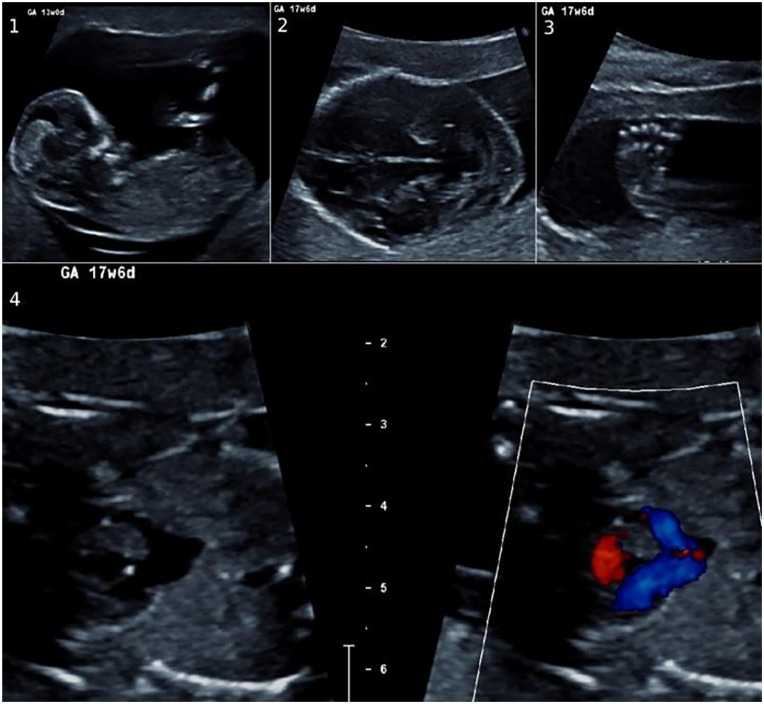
Fetus 1: ultrasound images. Fetus 1 with (1) elevated nuchal fold thickness, (2) absent cavum septum pellicidum, (3) club foot, and (4) functionally univentricular heart.
